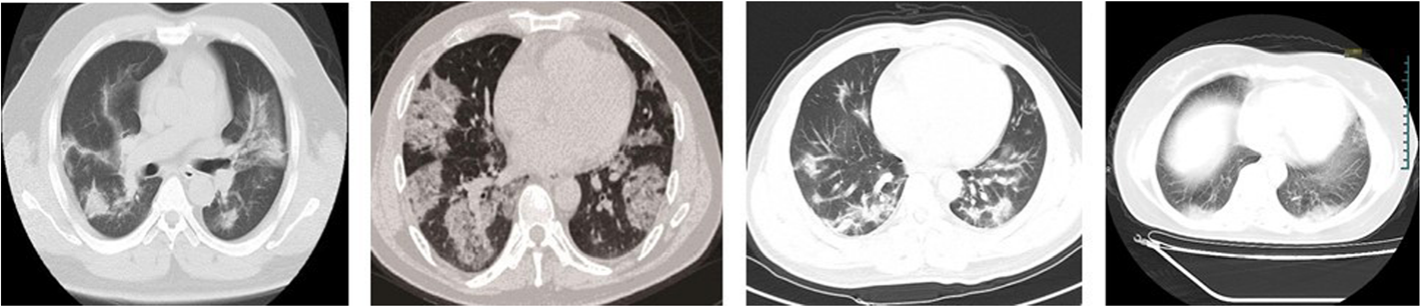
Sample COVID-19 CT images from the COVID-CT dataset
